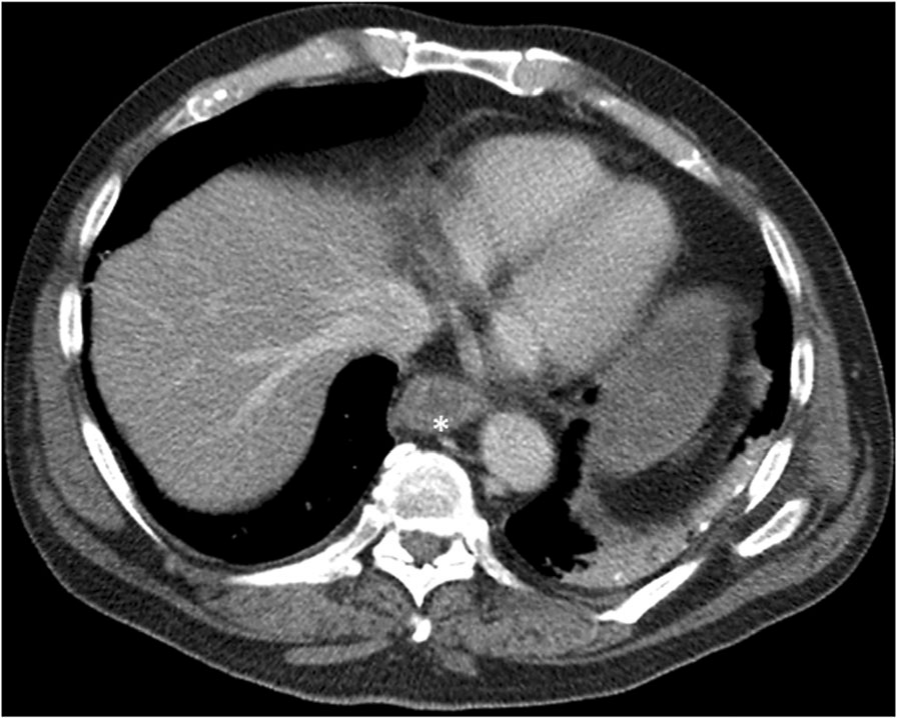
Computed tomography scan taken 12 hours after the patient ingested the wrong medication, showing an edematous distal esophagus (*) but no extraluminal air or inflammation
